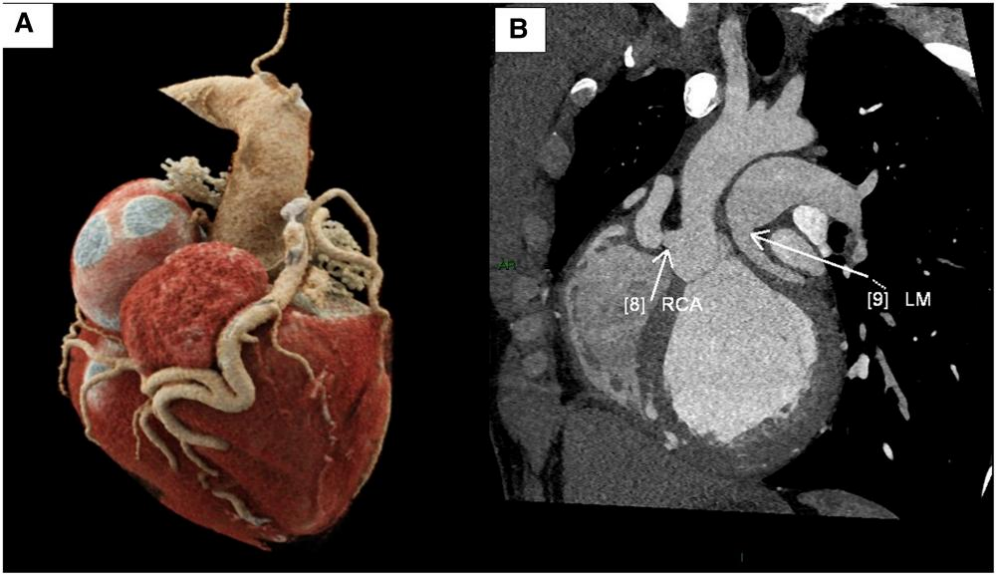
Computed tomography imaging of the coronary tree. (*A*) 3D rendering of the heart, showing the ectatic right coronary artery which supplies blood to the whole coronary tree. (*B*) A maximal intensity projection of the origins of the coronary arteries: the right coronary artery originating from the aorta and the left main originating from the left pulmonary artery.
